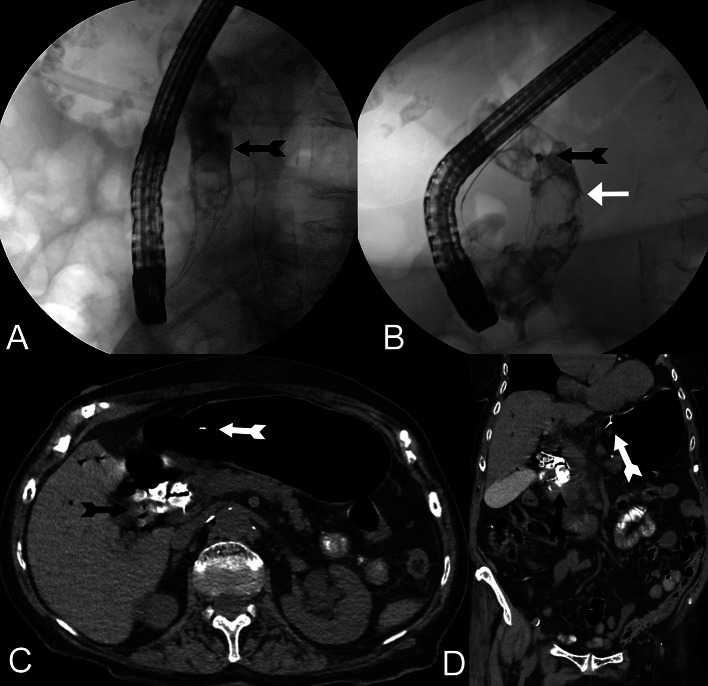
(A-D) (A and B) Second ERCP with the attempt to disintegrate and/or retrieve multiple bile stones in the CBD. Unfortunately, the gastroenterologists encountered a rupture of the DB (black feather arrow) with the pulling-wire (white arrow) (c and d) Contrast-enhanced follow-up CT after the second ERCP showed the severed DB (black feather arrow) impacted in the CBD with the pulling-wire reaching from the DB through the complete nasopharyngeal tract (white feather arrow).
